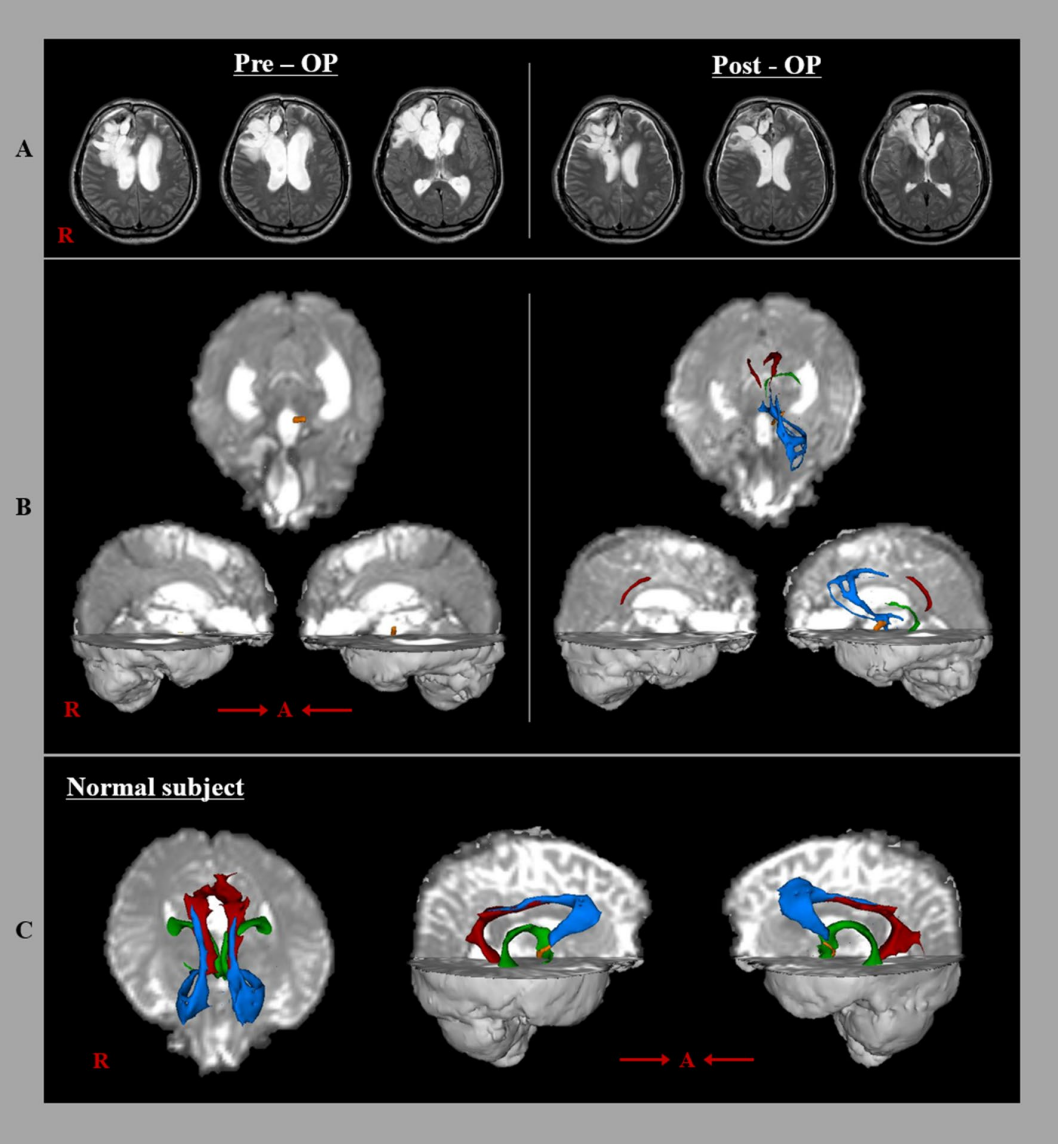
a The pre-operative T2-weighted brain magnetic resonance images show dilatation of the ventricular system, as well as improvement of dilatation of the ventricular system, after ventriculoperitoneal (VP) shunt installation. b Results of diffusion tensor tractography (DTT) for each neural tract of the Papez circuit of the patient: The left mamillothalamic tract (yellow) is reconstructed, whereas other neural tracts of the Papez tract are not reconstructed on pre-VP shunt DTT. By contrast, the left mamillothalamic tract is thickened, and the left thalamocingulate tract (sky-blue), fornix (green), and both cinguli (red) are reconstructed on post-VP shunt DTT. c Results of DTT for each neural tract of the Papez circuit of a normal subject (35-year-old male): thalamocingulate tract (sky-blue), fornix (green), cingulum (red), and mamillothalamic tract (yellow) (color figure online)
